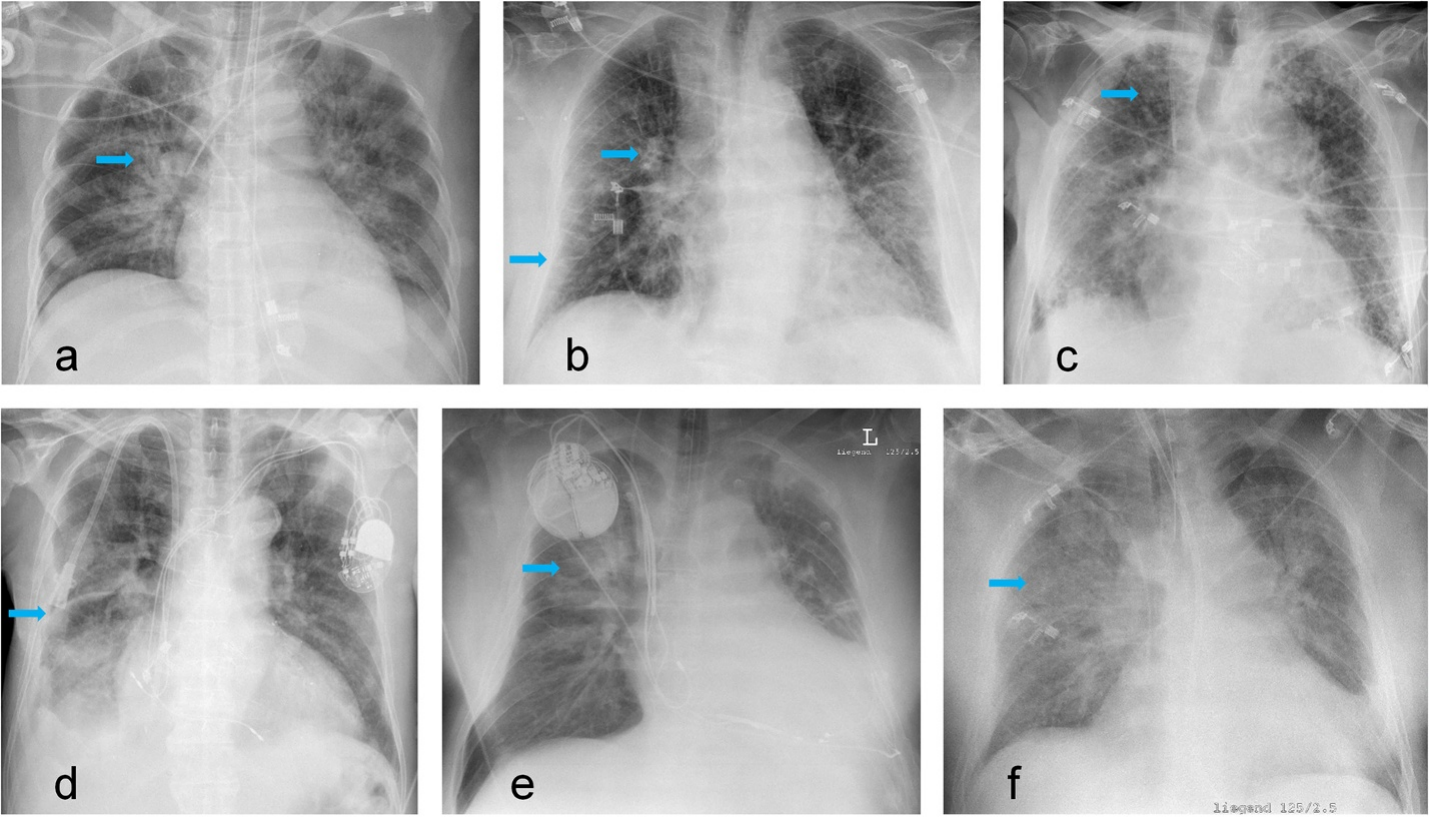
Bedside chest radiographs showing different indications of pulmonary edema. Enlarged/blurred hilar vessels (a), Kerley lines (lower arrow) and peribronchial/perivascular cuffs (upper arrow) (b), micronoduli (c) widening of interlobular fissure (d), extensive perihilar haze (e) and diffuse increase in density (f).
